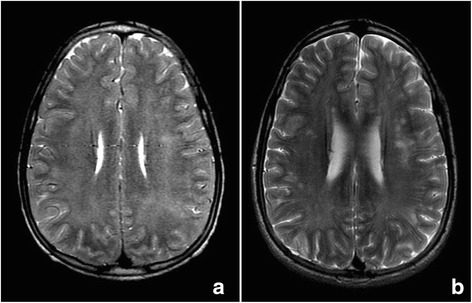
Axial section MRI T_2_-weighted sequences at 72 h. Image a indicates an increased intensity in white matter of diffuse bilateral distribution. Image b taken after 8 months; indicates resolution of the previous diffuse affectation, however the appearance of specific areas with increased signal in the periventricular brain parenchyma predominantly of the left side, indicative of definitive residual injury
